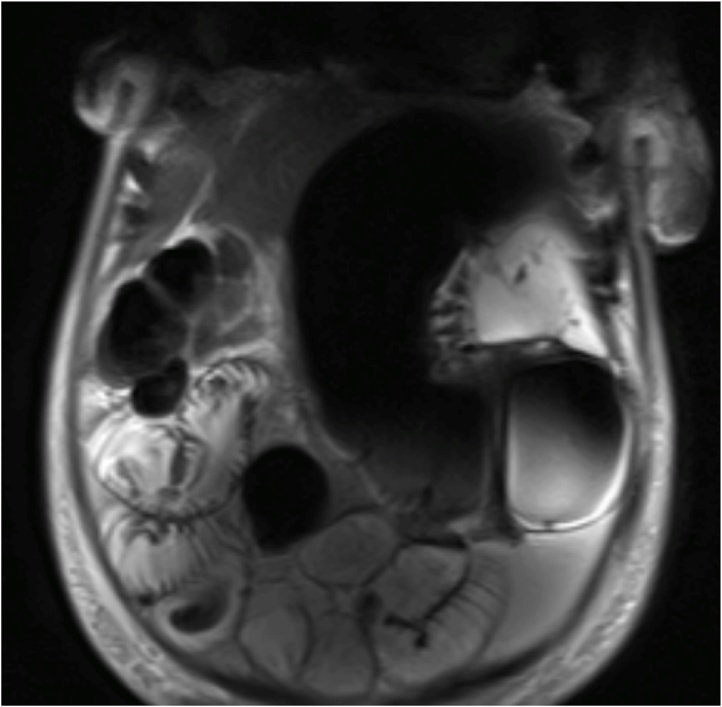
MRI abdomen, coronal view showing large bowel volvulus in the left upper quadrant.
